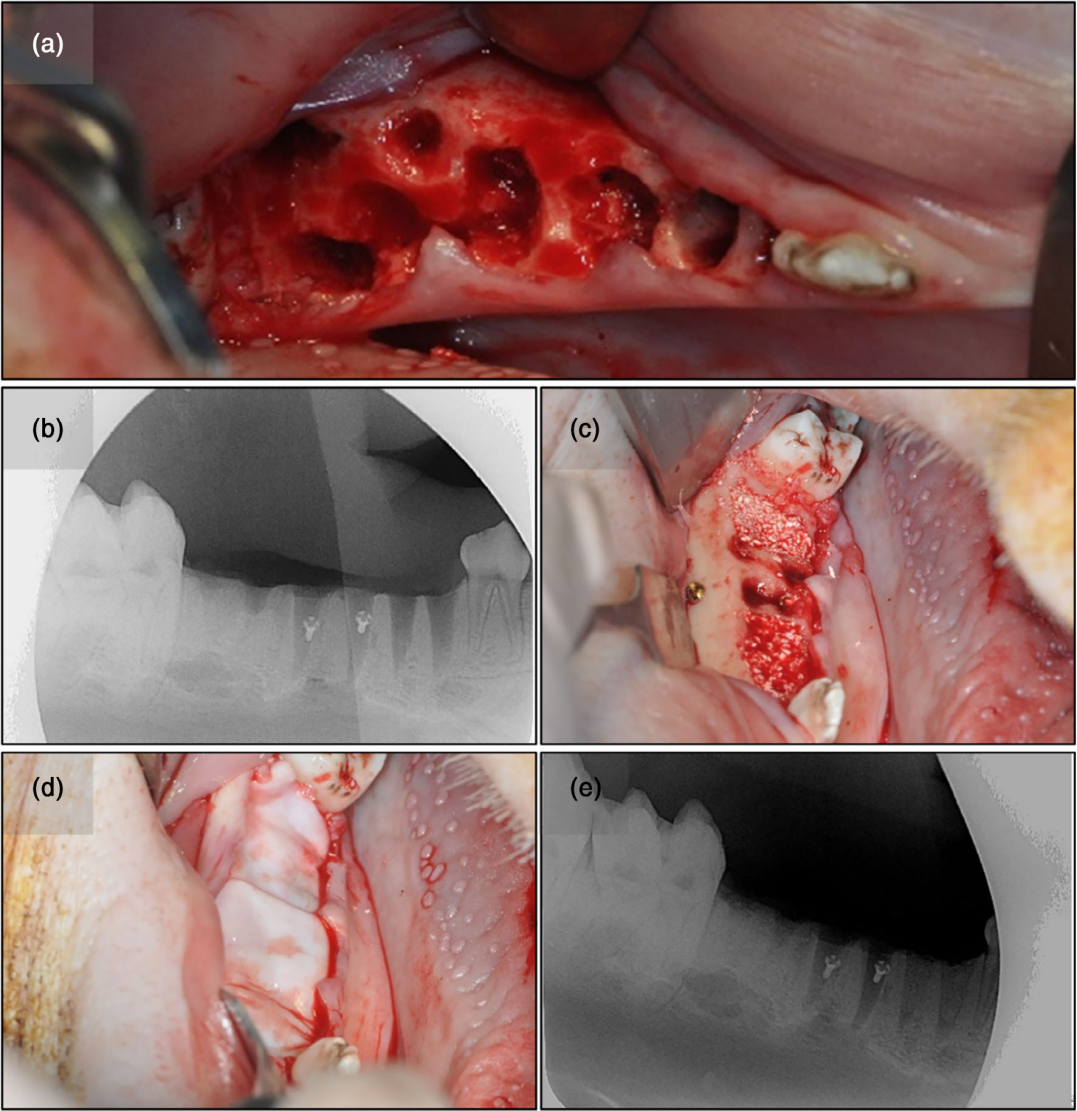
Clinical situation of an extraction site after removal of the third and fourth premolars and the first molar (a). Complete tooth removal was verified radiographically (b) before the defects were augmented with particulate biomaterial (c) and covered with a resorbable membrane (d). The postoperative radiograph (e) shows the alveolae filled with bone substitute material and osteosynthesis screws placed for orientation purposes
